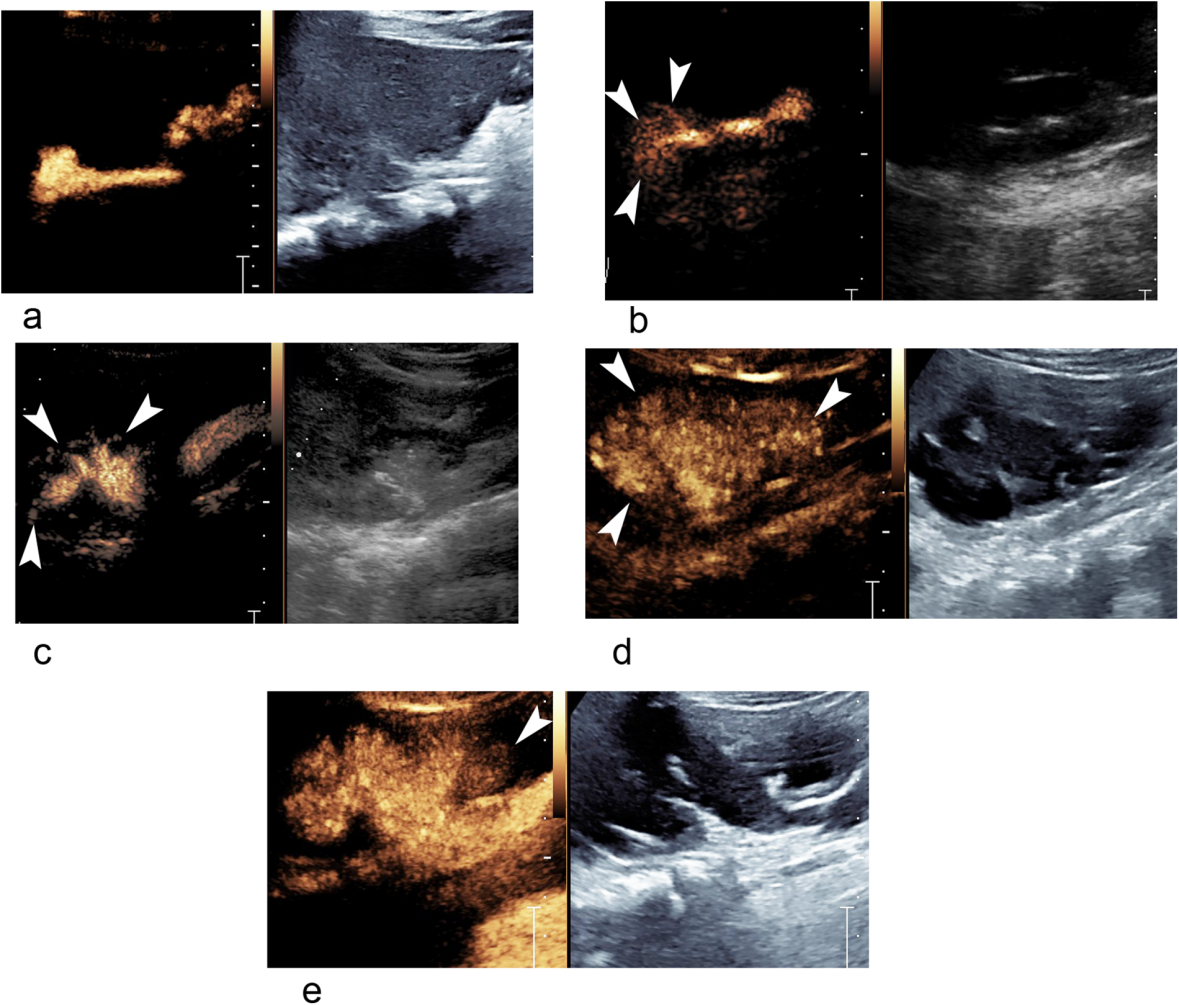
Different grades of VUR and IRR in ceVUS ultrasound images. (a) A 22-month-old boy with grade II VUR of right kidney without IRR. (b) A 6-month-old girl with grade II VUR and IRR (arrowheads) of right kidney. (c) A 5-month-old boy with grade III VUR and IRR (arrowheads) of right kidney. (d) A 13-month girl-old with grade V VUR and IRR (arrowheads) of right kidney. (e) The same patient as in (d), grade IV VUR and IRR (arrowhead) of left kidney. IRR, intrarenal reflux; ceVUS, contrast-enhanced voiding urosonography; VUR, vesicoureteral reflux.
